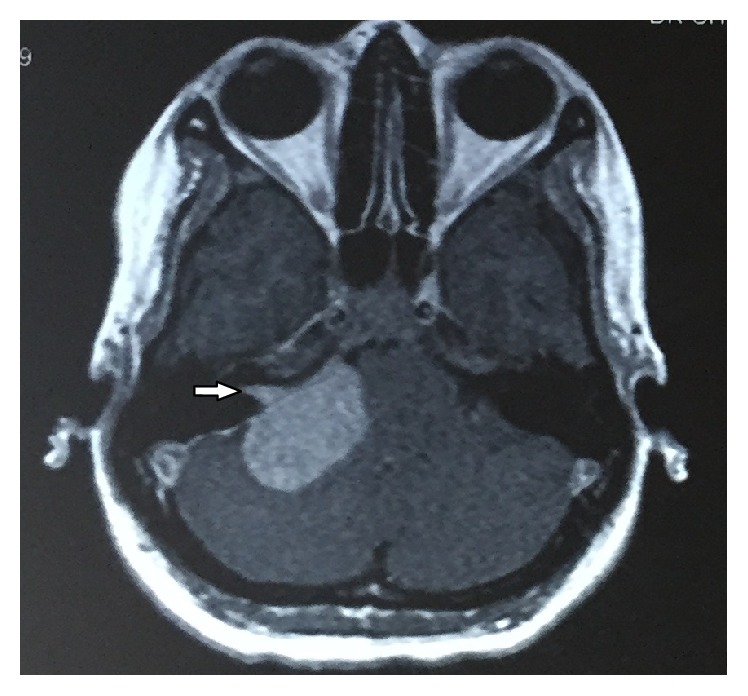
Contrast axial MRI shows the extension of the tumor into the internal auditory canal represents “shark fin” appearance (white arrow).
